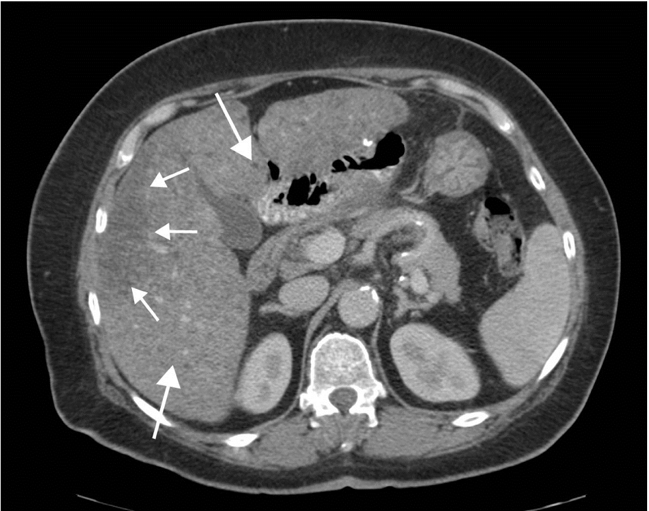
Upper abdominal CT examination in a patient approximately three months after bariatric gastric bypass, performed for upper abdominal pain. There are abnormal liver appearances with multiple small well defined areas of low attenuation (long arrows) together with a larger more confluent area with a typical ‘geographical’ appearance peripherally in the right lobe (short arrows). These changes were due to patchy fatty infiltration.
